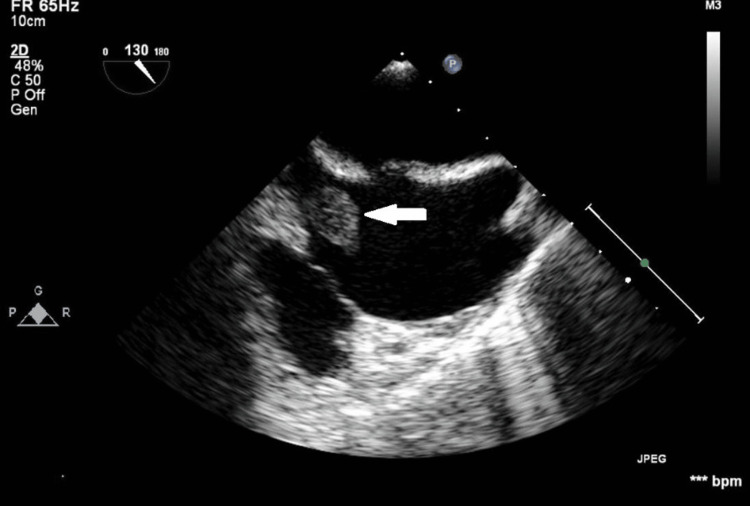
TEE view at approximately 115 degrees, focusing on RA showing a round-shaped mass (white arrow) TTE, transthoracic echocardiogram; RA, right atrium
